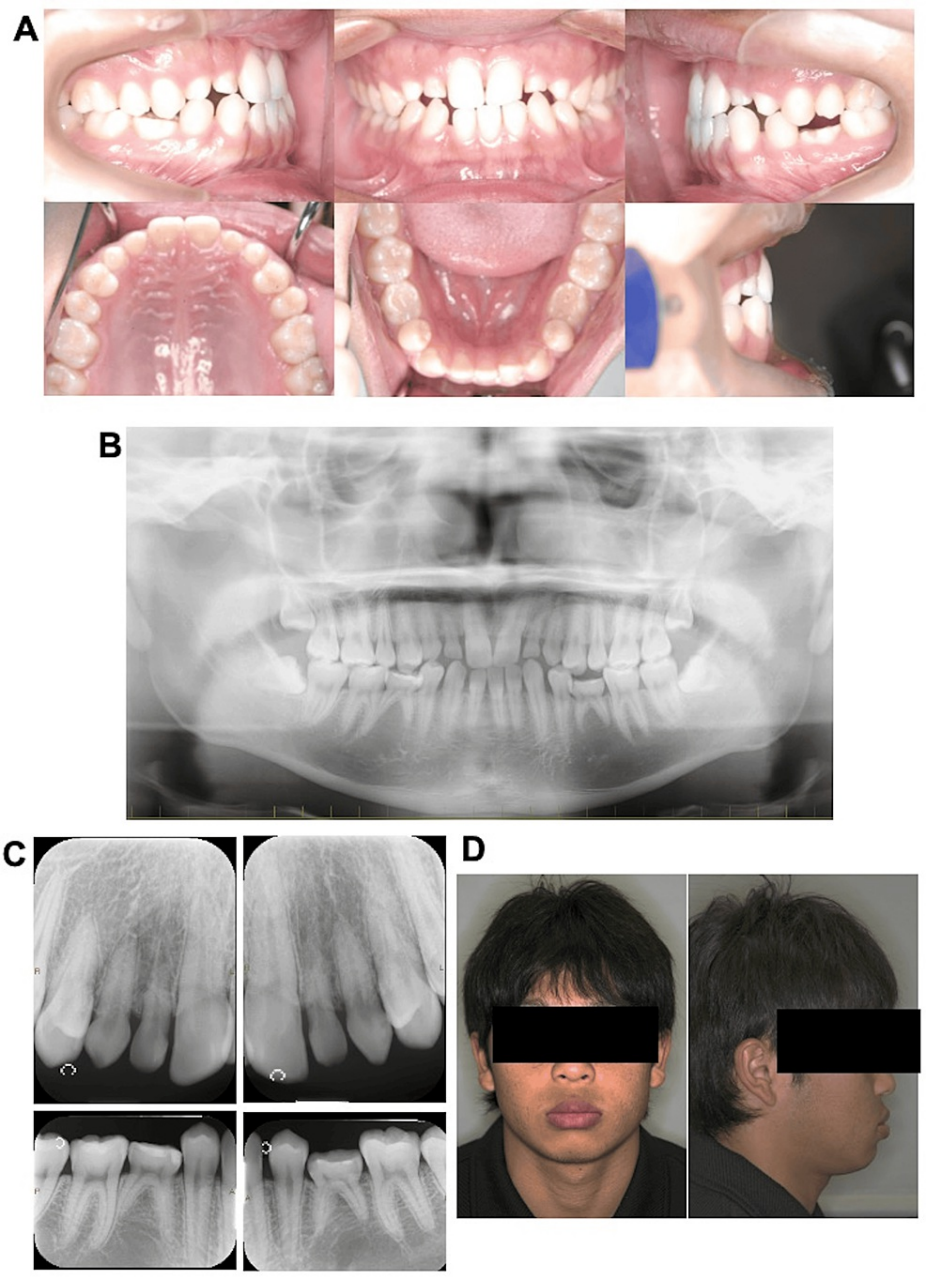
Initial examination A: pretreatment intraoral photographs; B: pretreatment panoramic radiograph; C: pretreatment intraoral radiograph; D: pretreatment facial radiograph
